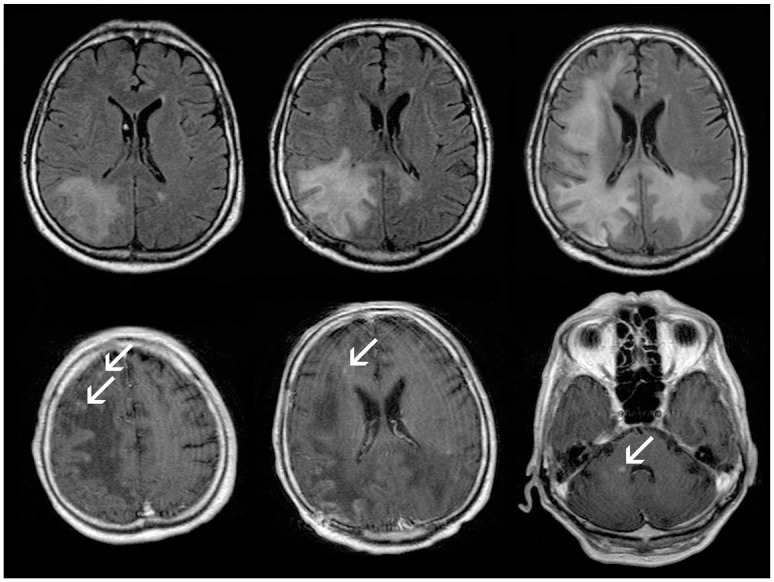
Follow-up (within 2 months) axial T2-FLAIR (upper row) and axial contrast enhanced T1-SE (lower row) in patient 2, extensive progression of posterior accentuated, mostly subcortical PML-lesions is observed (upper row). Approximately 3 months after onset of the neurological symptoms, punctuate contrast enhancement (arrows) appear at the borders of the PML lesions suggesting the development of IRIS, corresponding to further clinical deterioration (lower row). IRIS, immune reconstitution inflammatory syndrome; PML, progressive multifocal leukoencephalopathy.
